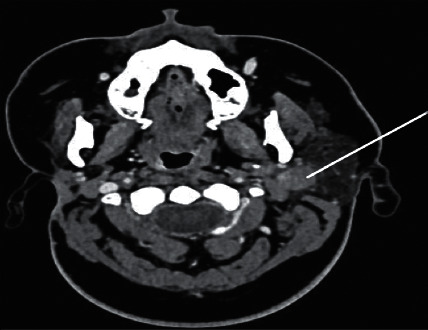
The CT scan showing a left deep parotid gland lesion in 2020.
